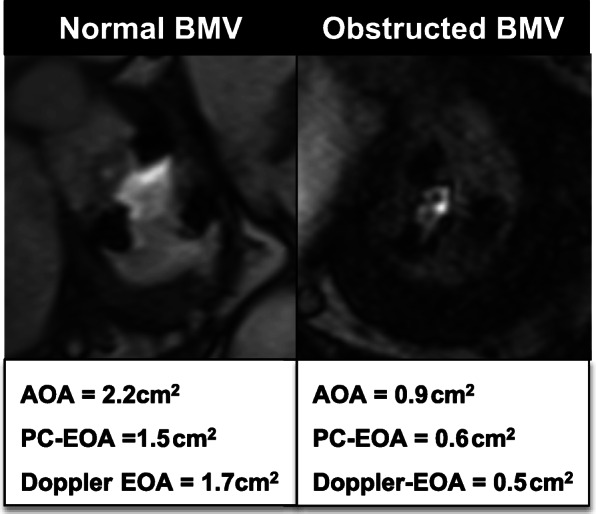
Clinical examples of normal and obstructed bioprosthetic valves assessed by CMR. A 65 year old male with a 29 mm bioprosthetic mitral valve. Phase contrast effective orifice area (PC-EOA) and DopplerEOA were similar and within normal range (left). A 60 year old male with dyspnea and a 25 mm bioprosthetic mitral valve. PC-EOA and DopplerEOA were both severity reduced, consistent with severe prosthetic valve stenosis (right)
